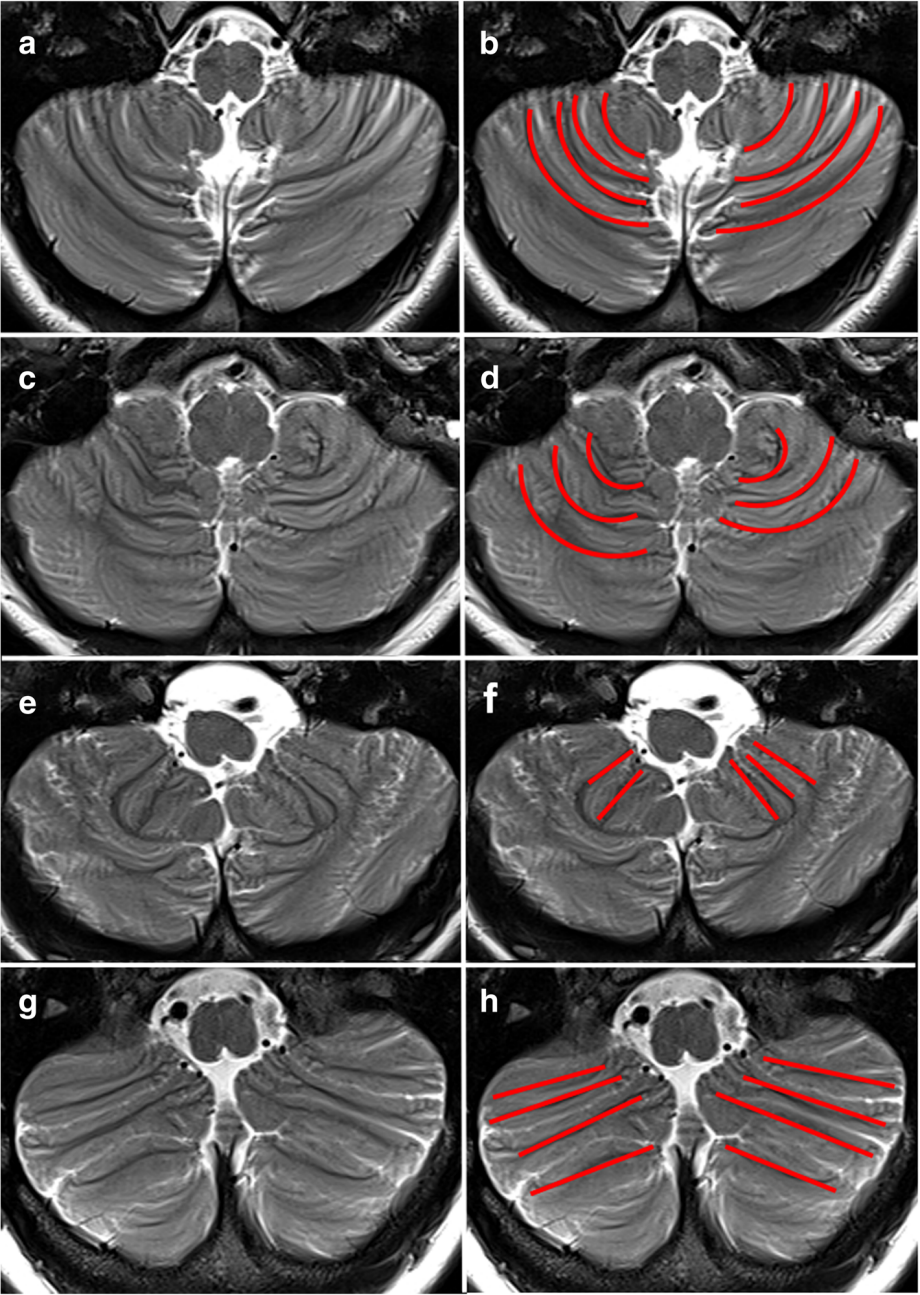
Brain MRI, axial T2-weighted images through the lower cerebellum of a normal subject (a, b) and three WHIM patients (c-h). The same MR image is shown in each row: the MR image shown on the right column is presented with an overlying scheme of the foliation pattern (red lines). Panels a, b Normal subject: the cerebellar folia show a curvilinear course from posteromedial to anterolateral (“onion-like” configuration). Panels c, d. WHIM patient (P1) with normal “onion-like” orientation of the cerebellar folia. Panels e, f: WHIM patient (P5): the cerebellar folia show a straight course from posterolateral to anteromedial in a well ordered parallel pattern, confined to anterior portion of the cerebellar hemispheres. Panels g, h.WHIM patient (P4): the abnormal straight course of the cerebellar folia is more extensive, involving the whole hemispheres
